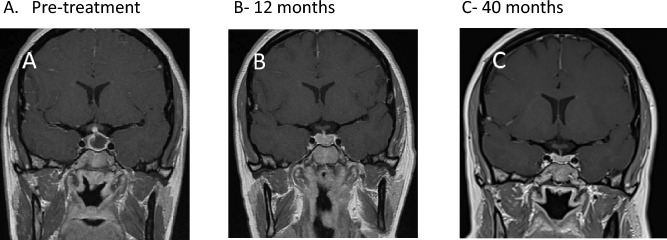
Coronal post-contrast T1W MR images of the pituitary. A Pre-treatment scan MRI pituitary showing a cyst-like peripherally enhancing pituitary lesion and a thickened pituitary stalk. There is distortion of the optic chiasm, but no compression. B Image following 6 months of treatment, MRI pituitary showing minimal residual enlargement of the pituitary gland. Resolution of the cyst-like component. Normal appearing pituitary stalk and optic chiasm. C 40 months of treatment, image showing radiologically normal appearing pituitary
